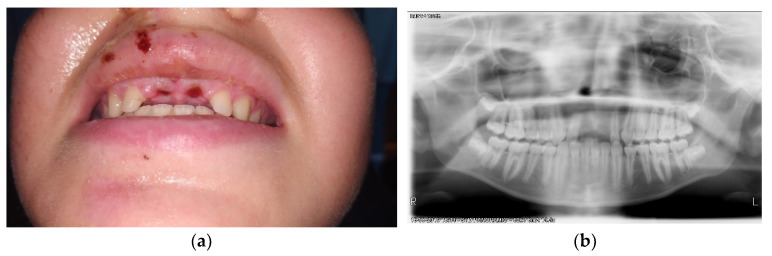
(a) Orofacial trauma with upper central incisors missing; (b) Panoramic X-ray confirming avulsion of teeth 11 and 21.
